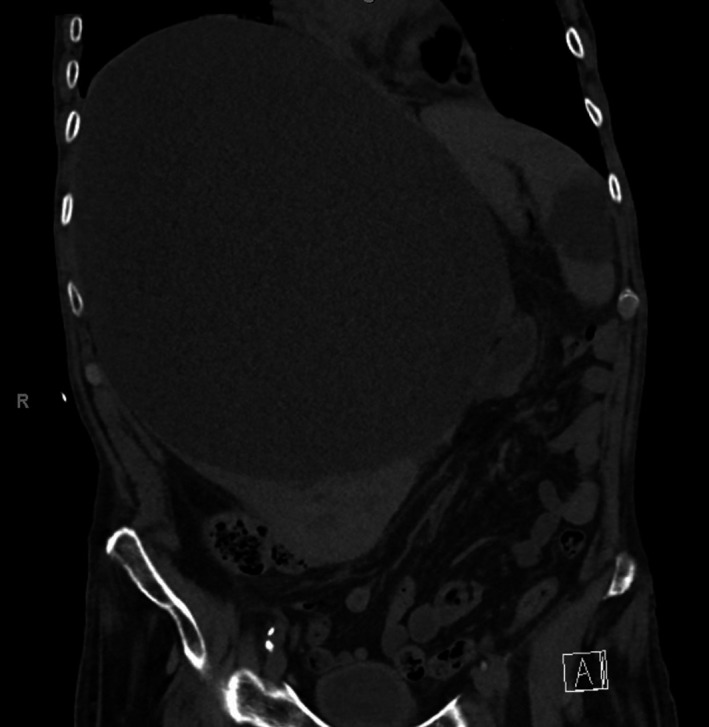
Computed tomography of the abdomen, coronal section: Huge liver cyst with displacement of the upper abdominal organs, gastrointestinal tract, and intra‐abdominal vessels
